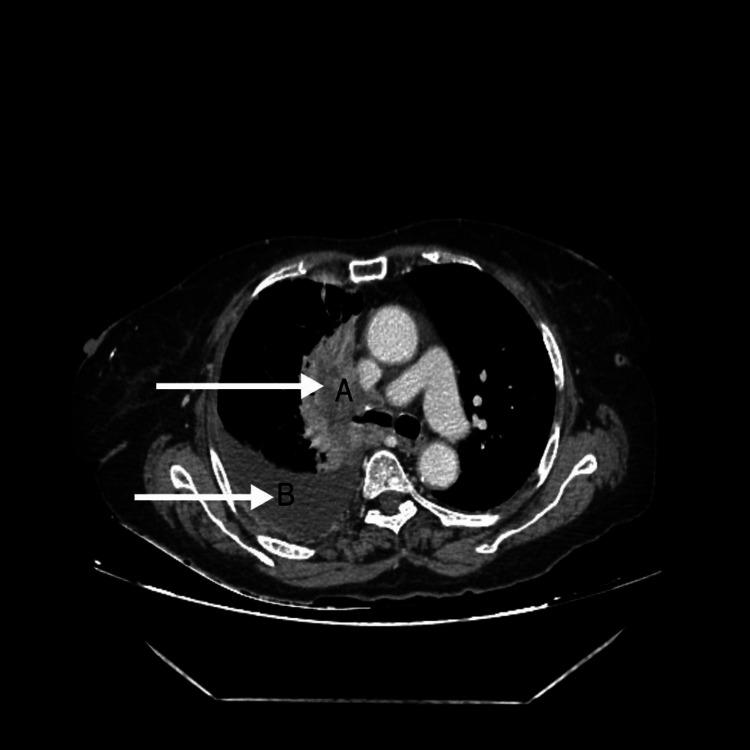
Computed tomography of chest showing right hilar mass with large discrete conglomerated lymph node measuring 71 x 39 mm in right hilar region with right pleural effusion A: Right hilar mass with enlarged hilar lymph node; B: Right-sided pleural effusion
